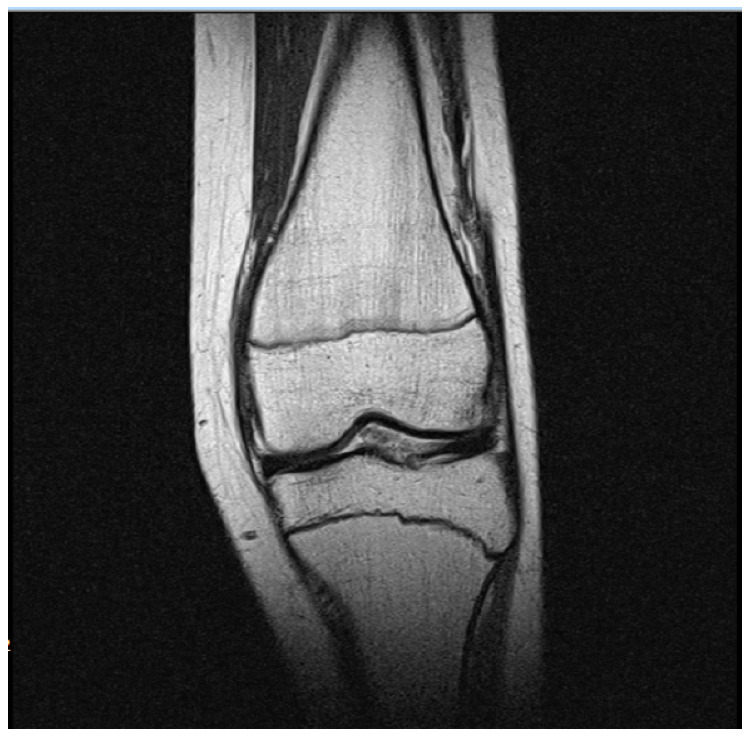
Original DICOM image of the patient’s knee from MRI T2WI.
